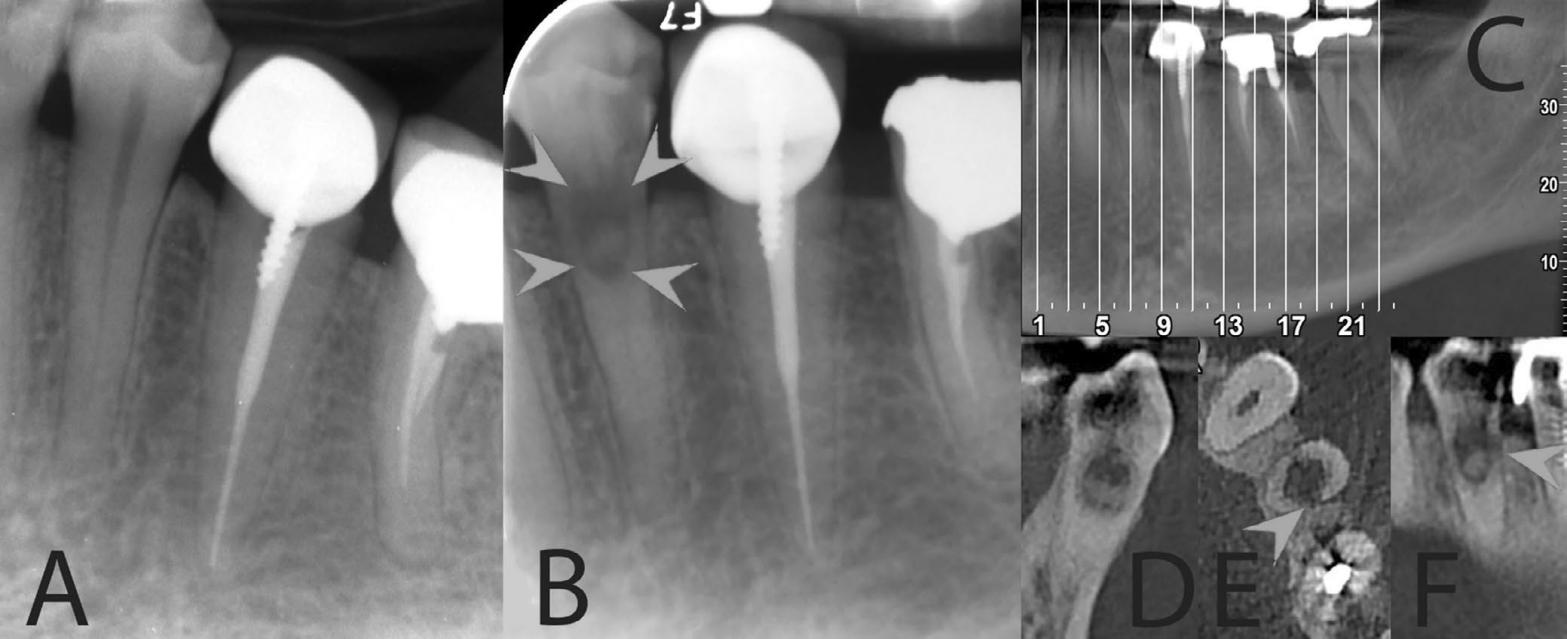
Radiographic and CBCT Images of Tooth #34. (A) Initial radiograph of the left first premolar taken 5 years prior, showing no signs of resorption or pathology. (B) Diagnostic periapical radiograph displaying a deep distal carious lesion and a significant resorptive defect in the coronal portion of the root of tooth #34 (white arrow heads). (C–F) CBCT images (in coronal, axial, and sagittal plans) of tooth #34, showing a large resorptive lesion extending into the midroot, with a distal perforation (white arrow heads) covered by surrounding bone. The lesion's extent is clearly demonstrated, aiding in accurate diagnosis and treatment planning.
